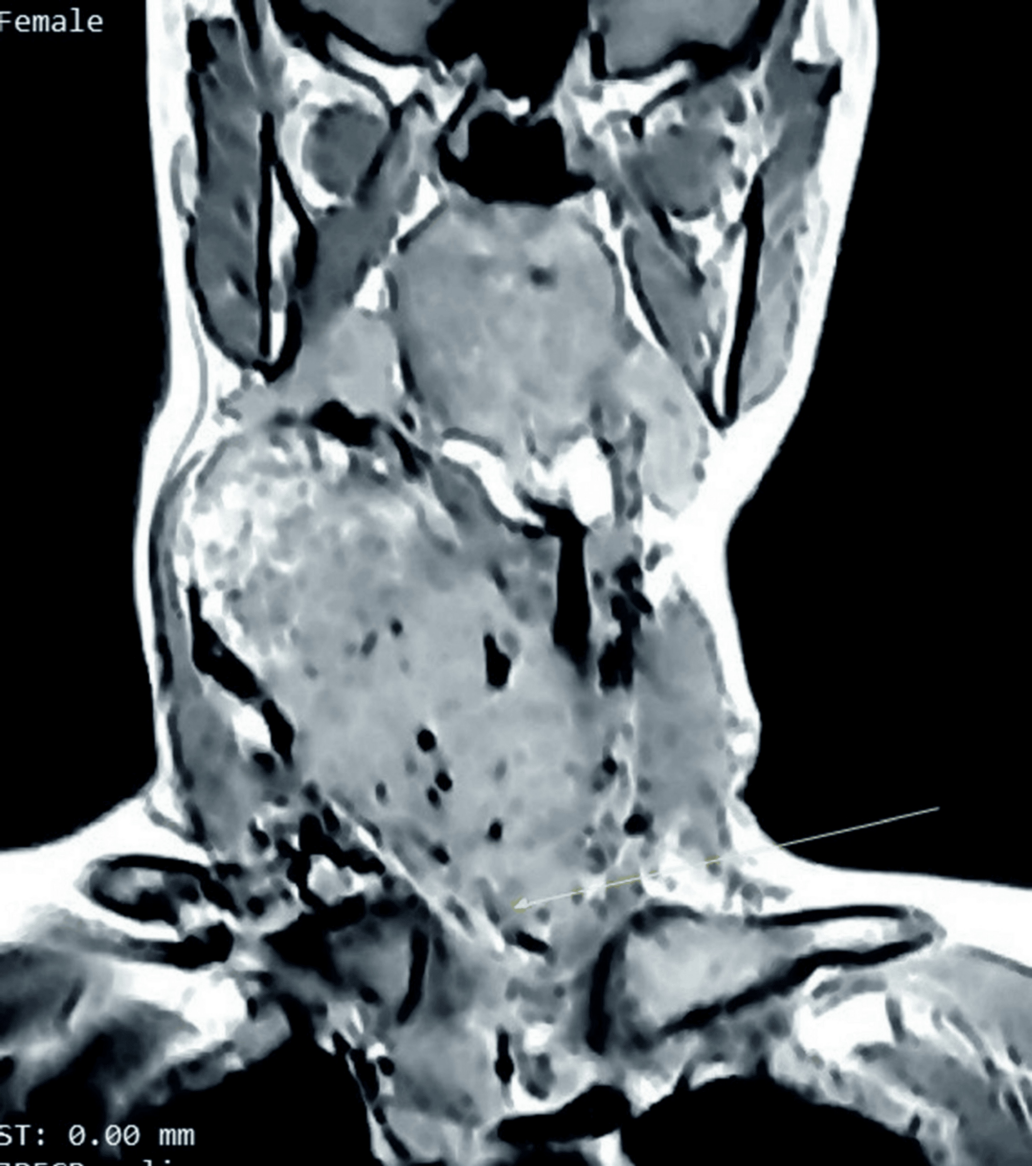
MRI of the neck in a 35-year-old female showing an enlarged thyroid gland with retrosternal extension. The yellow arrow indicating the retrosternal extension.
